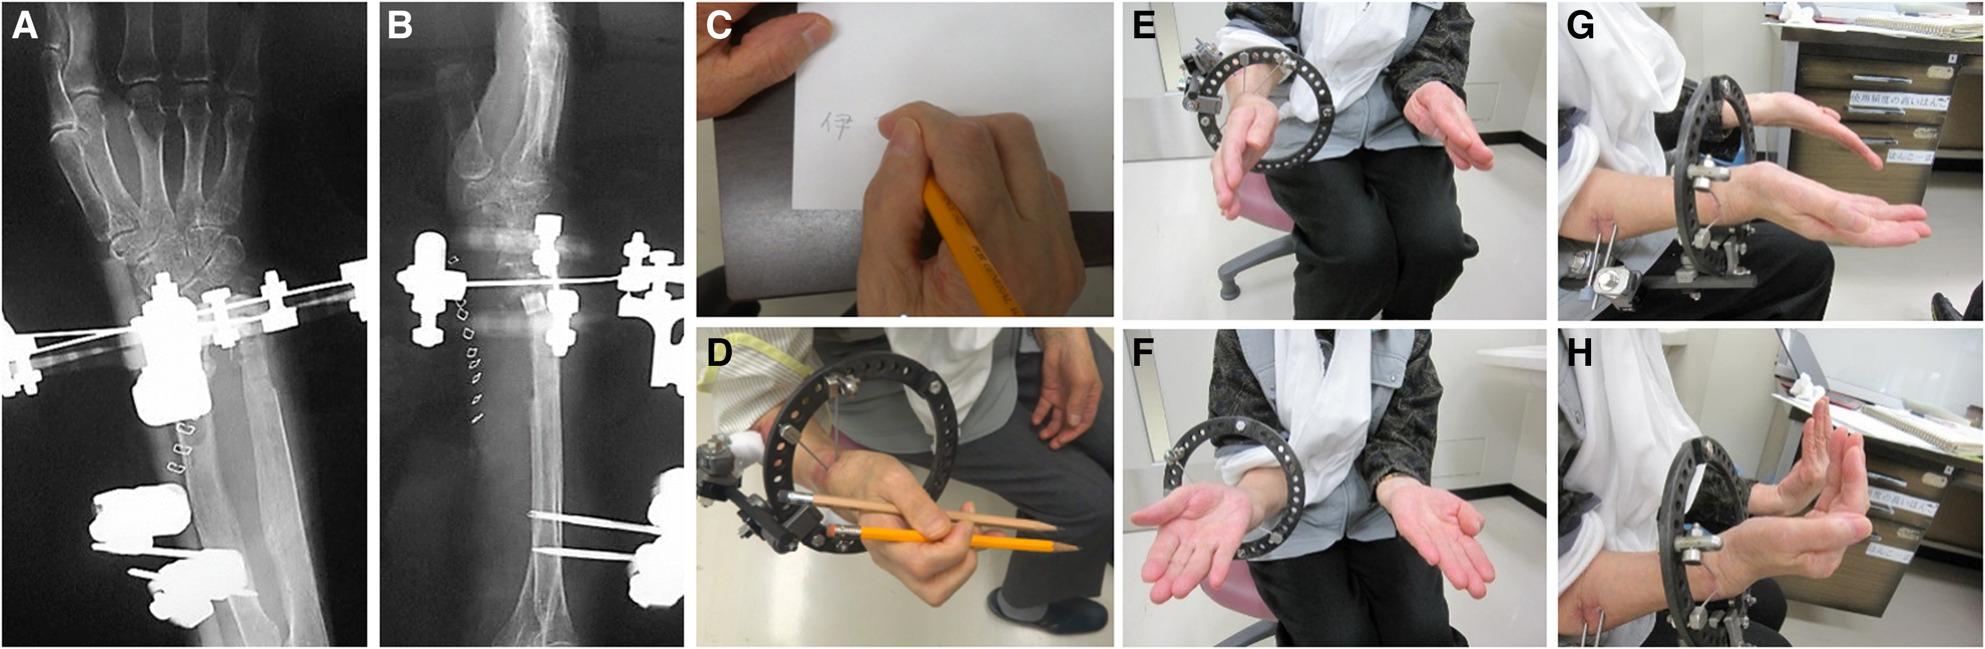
Clinical and radiographic findings after first-stage surgery. A Immediate postoperative anteroposterior radiograph showing non-bridging circular external fixation. B Immediate postoperative lateral radiograph. C Writing activity performed during external fixation. D Chopstick use during external fixation. E Active forearm pronation. F Active forearm supination. G Active wrist dorsiflexion. H Active wrist palmar flexion
